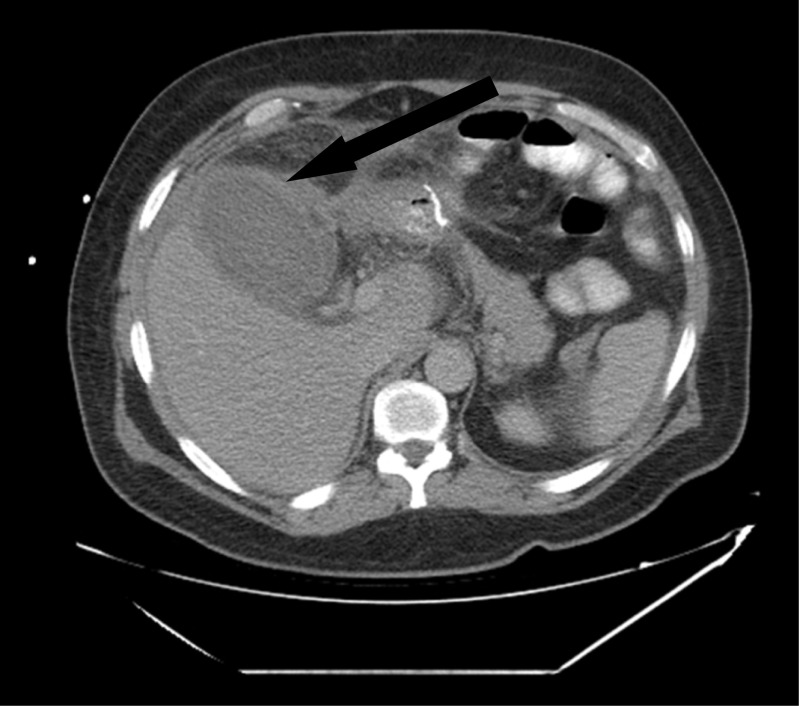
CT scan showing acute cholecystitis
